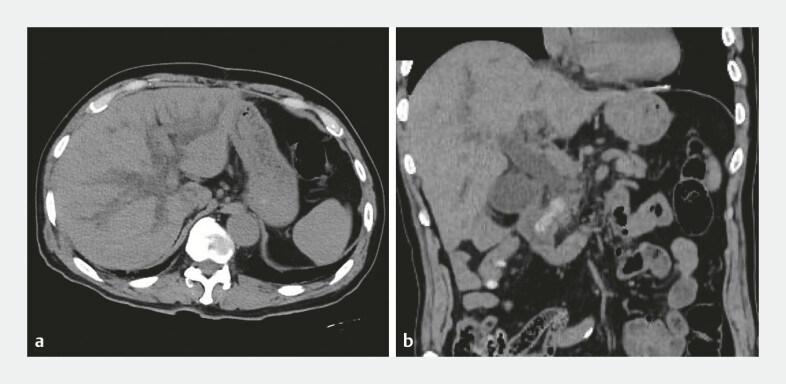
Pre-procedural computed tomographic images.
